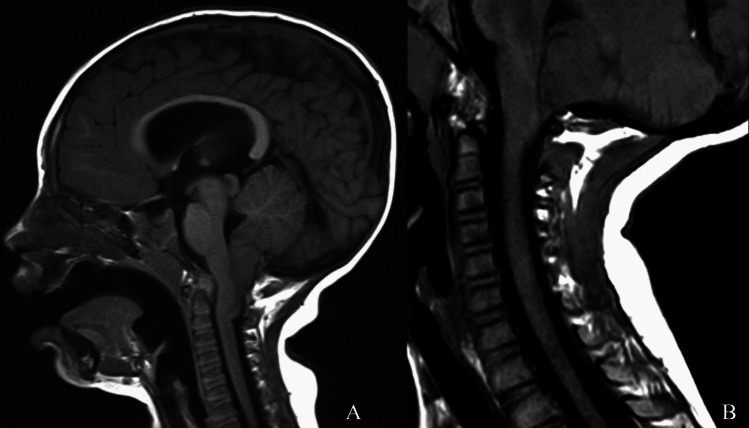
Pre-operative MRI brain (A) and post-operative MRI C-Spine (B) in a patient with Crouzon syndrome and Chiari I who underwent posterior vault distraction osteogenesis with concurrent extradural Chiari decompression. Pre-operative tonsillar herniation was 13 mm. At 29 months post-operatively, tonsillar herniation was improved at 6 mm
